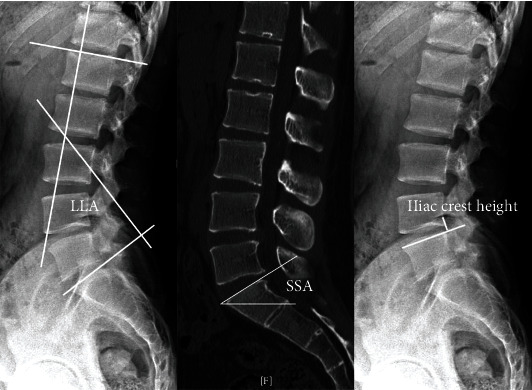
Measurement of LLA, SSA, and iliac crest height. SSA: sacral slope angle; LLA: lumbar lordosis angle; iliac crest height (we measured the distance on lateral radiographs between the upper margin of the L5 vertebrae and the highest point of the iliac crest as the iliac crest height).
